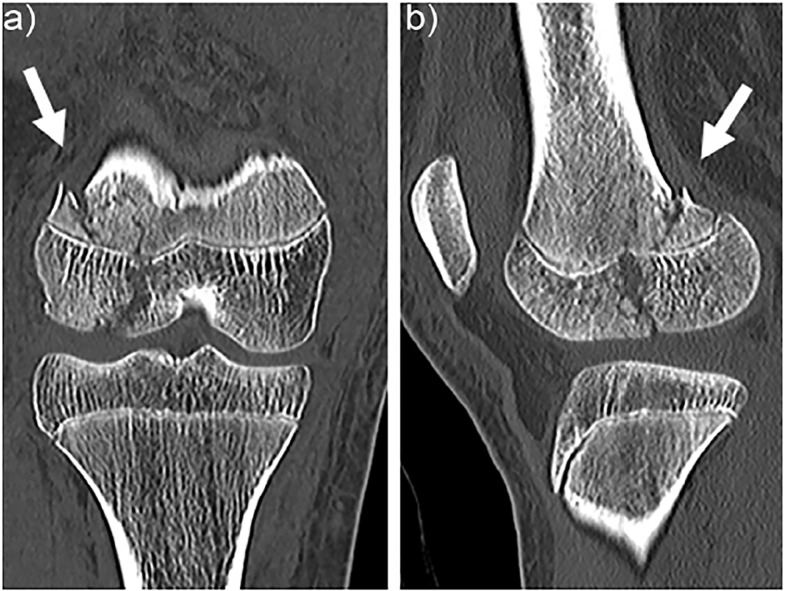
Coronal (A) and sagittal (B) views on computed tomography show injury to the distal femoral epiphyseal growth plate (Salter-Harris type 4 and tenderness at the point near the epiphyseal closing) (arrow).
